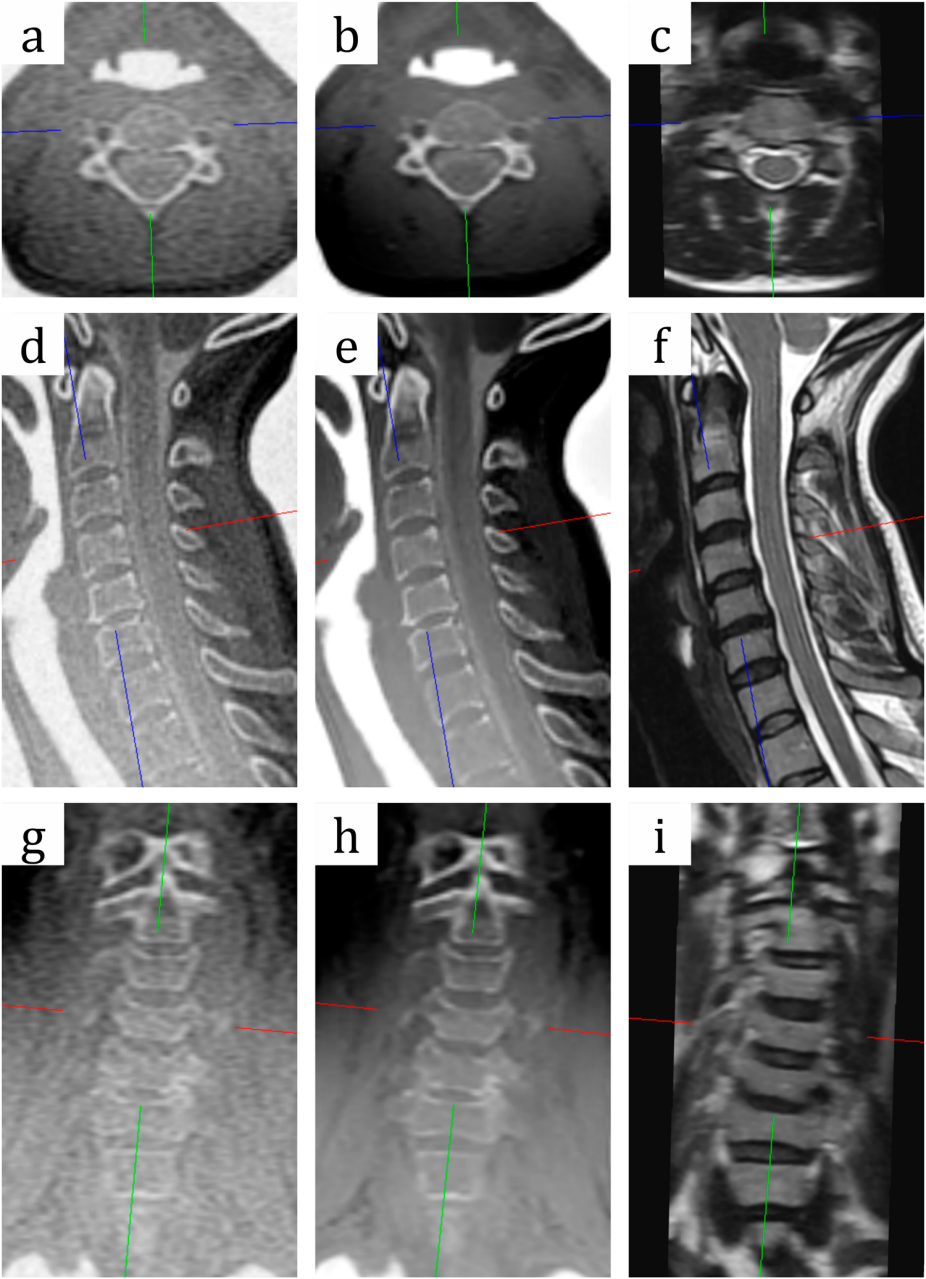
MR images of a 28-year-old female patient with cervical pain after trauma 3 months previously. Multiplanar reconstructions of the cervical spine with axial (a–c), sagittal (d–f), and coronal oblique (g–i) planes. ZTE sequences (a, d, g), ZTE-DL sequences (b, e, h) and T2w sequences (c, f, i) demonstrate minor spondylotic changes at the level of C5/6 with narrowing of intervertebral space and discrete anterior and posterior osteophytes at the endplates of C5/6
